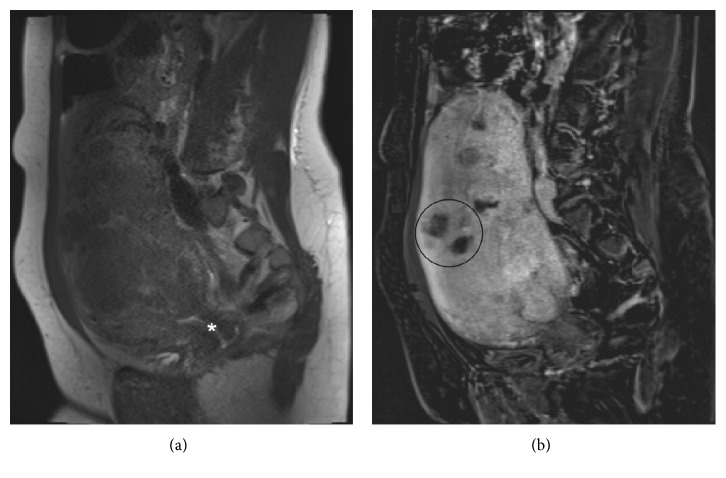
Sagittal view of pre-UFE pelvic MRI (a) prior to and (b) after gadolinium contrast injection. An enlarged uterus, at the level of the cervical canal (asterisk), contains numerous fibroids, with heterogeneous enhancement. One such midanterior mass is annotated (circle).
